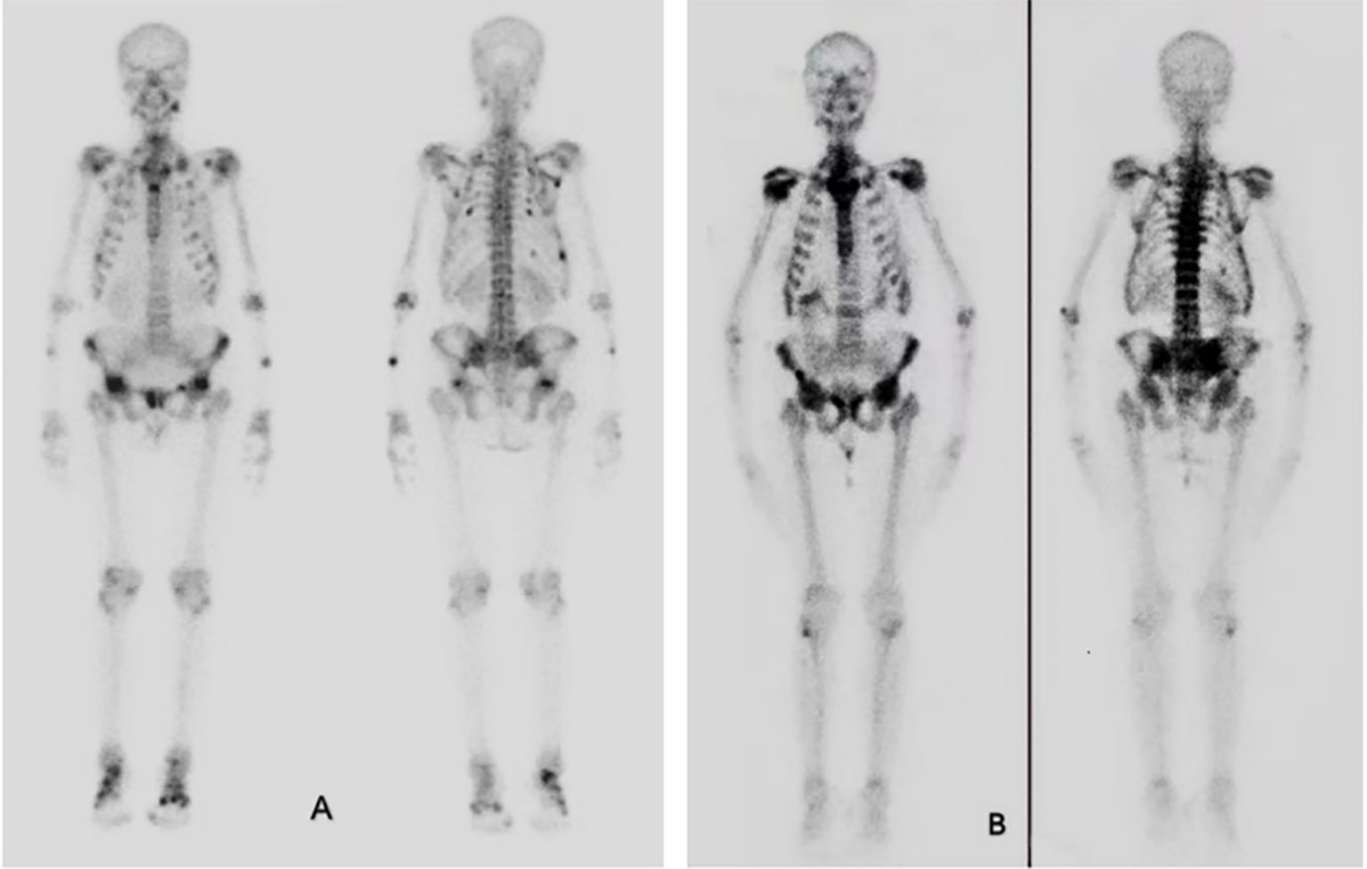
Bone scintigraphy. (A) Bone scintigraphy 10 years ago showed increased metabolism in whole body bones and joints, and increased spot-like metabolism in ribs, clavicle and pelvis, which was consistent with osteomalacia. (B) Bone scintigraphy in 2023 showed obvious concentration of axial bone nuclides in the whole body, with typical necktie sign and beaded rib changes. Nuclide concentration was evident in shoulder joints, pelvis, sacroiliac joints and spine.
